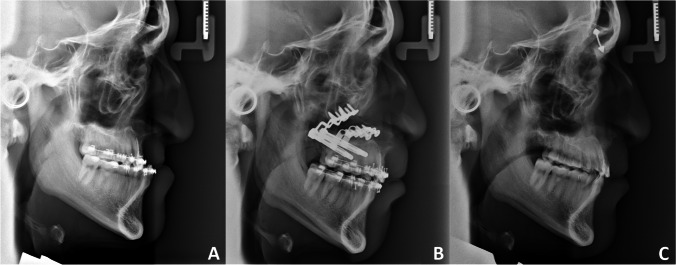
Radiographical examinations of the facial skeleton before and after treatment with DO. A, Lateral cephalogram prior to surgery (T0). B, Lateral cephalogram after surgery and advancement with the distraction device, at the start of the consolidation phase (T1). C, Lateral cephalogram at a mean of 18 months post-surgery (T2)
